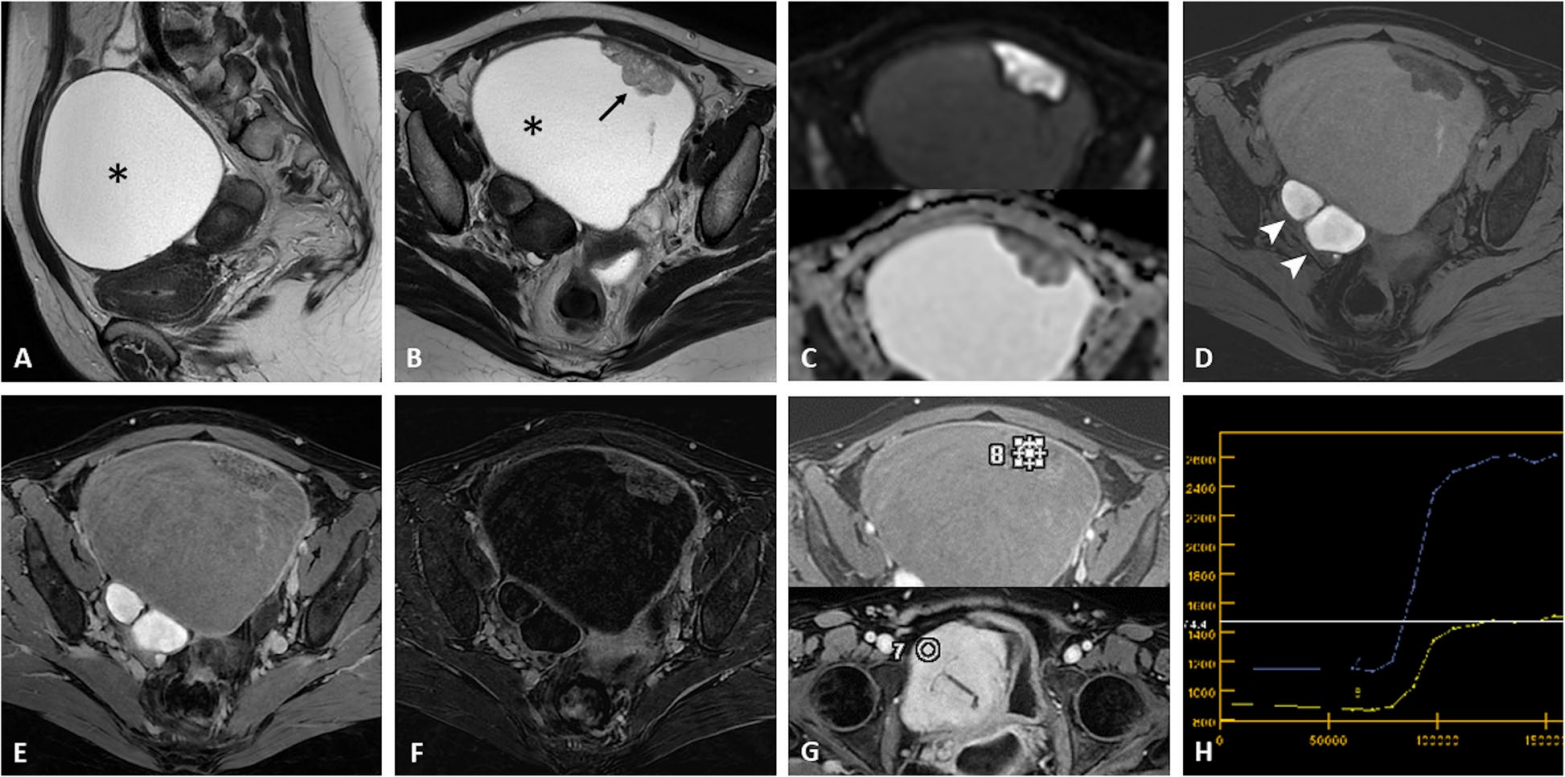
Ovarian endometrioid adenocarcinoma within an endometrioma in a 39-year-old woman with a known history of endometriosis. Sagittal T2-weighted image (a) shows a left ovarian cystic mass (asterisk) without a T2-shading effect. Axial T2-weighted image (b) shows a mural nodule (arrow) with restricted diffusion on a high *b800* value image and corresponding ADC map (c). The axial fat-suppressed T1-weighted image (d) displays a typical right ovarian endometrioma (arrowheads). Axial contrast-enhanced fat-suppressed T1-weighted image (e) shows a light enhancement of the nodule, better displayed on the subtraction sequence (f). Dynamic contrast enhancement analysis of the nodular portion (g, h) demonstrates a type 3 curve (blue curve, located above normal myometrium in the yellow curve), consistent with a malignant etiology
